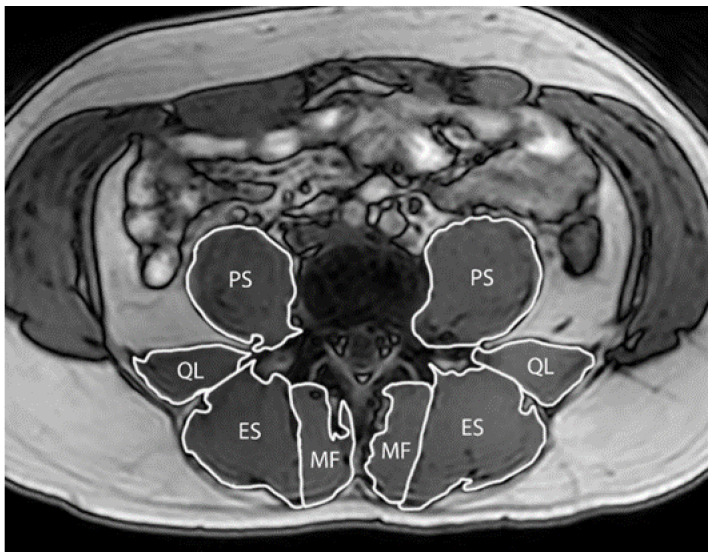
T2-weighted MRI image of the lumbar spine at the L4 vertebrae, showing traces of the multifidus (MF), erector spinae (ES), quadratus lumborum (QL) and psoas major (PS).
